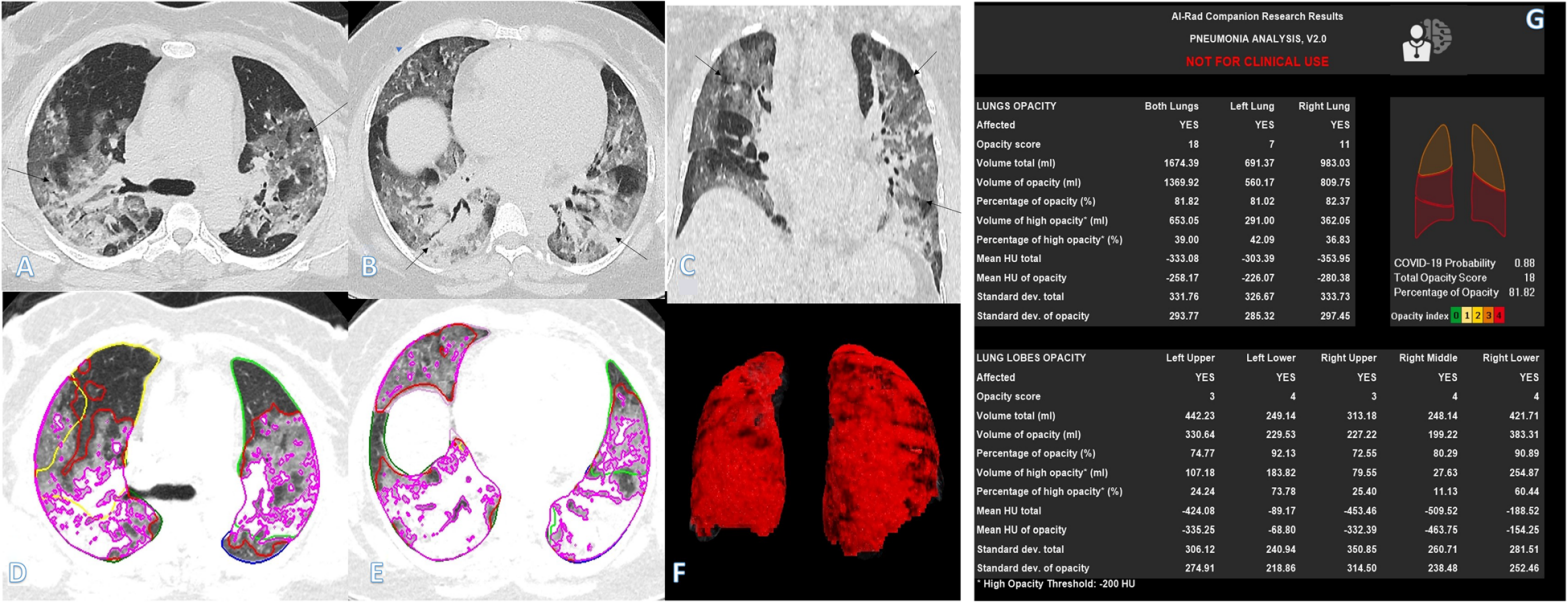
A 37-year-old female with positive COVID-19 virus. CT chest shows diffuse bilateral consolidation with air bronchogram, crazy-paving appearance, and organizing pneumonia pattern of COVID-19 (arrows in a–c). Total severity score = 18. Quantitative analysis (d–g) by AI-Rad Companion Research CT Pneumonia Analysis was presented with the measured parameters seen in table (g). Quantitative total opacity score was 18
